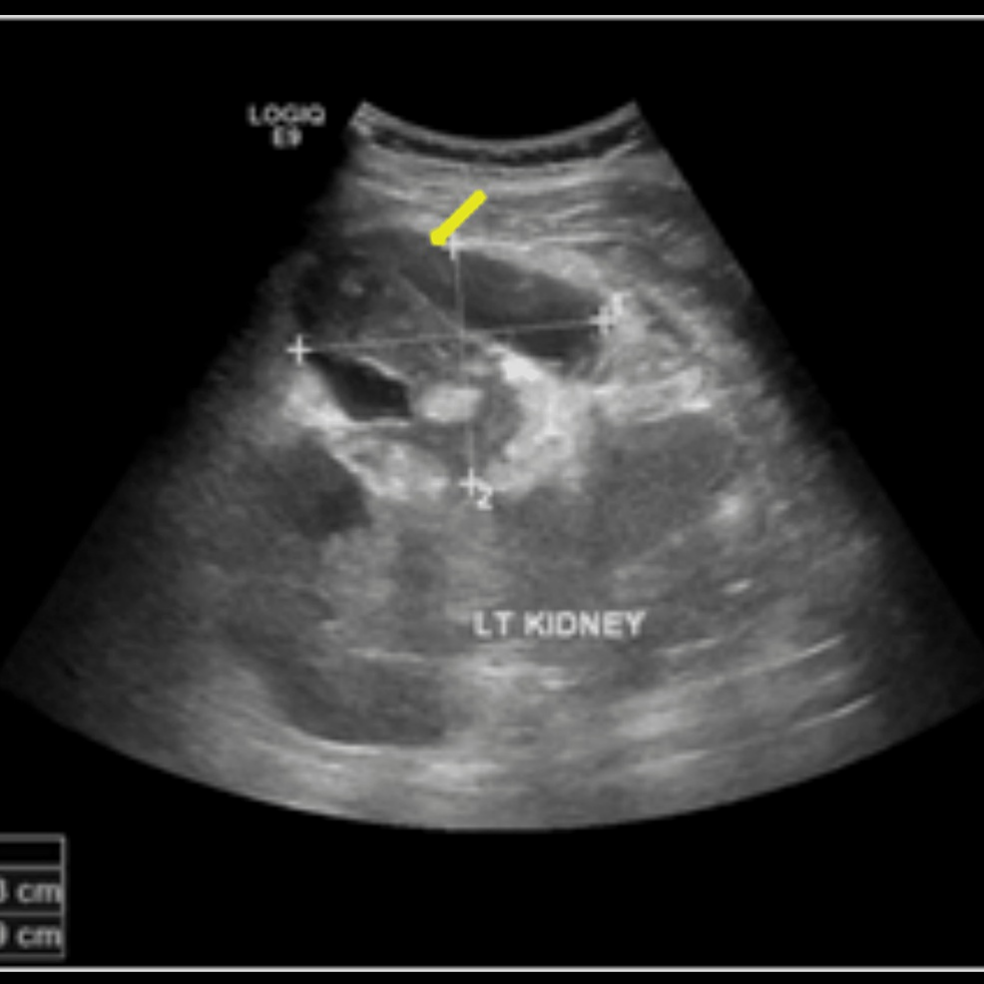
Ultrasound urinary tract showing a heterogenous cortical lesion at the interpolar region of the left kidney (yellow arrow).
